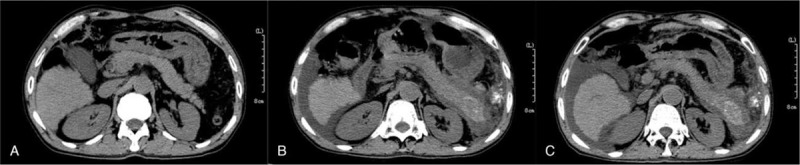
Abdominal CT image showing the pancreatic tail pre-and post-TACE. A: Normal pancreatic tail before TACE. B: Swelling and a low-density area in the tail of the pancreas, suggesting necrosis. Dense lipiodol accumulation in the dorsal pancreatic tail after the 3rd day of TACE. C: A flocculous and linear high-density shadow also appeared in the pancreatic tail, with a slightly low-density fluid effusion and a few spots of high density after 28th day of TACE.
